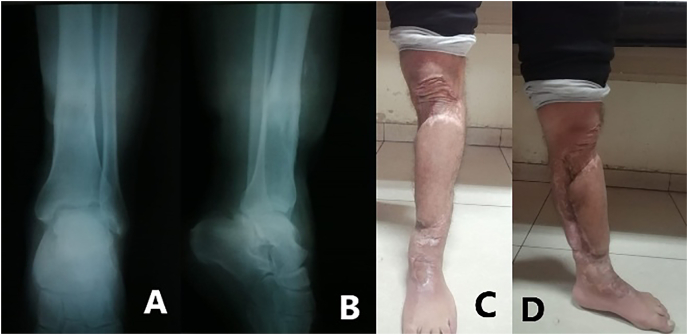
A year after; Anterior (A) and lateral (B) tibial X-ray show a healing tibial fracture. Anterior (C) and lateral (D) tibial clinical photographs show the flap and grafts were healing successfully.
